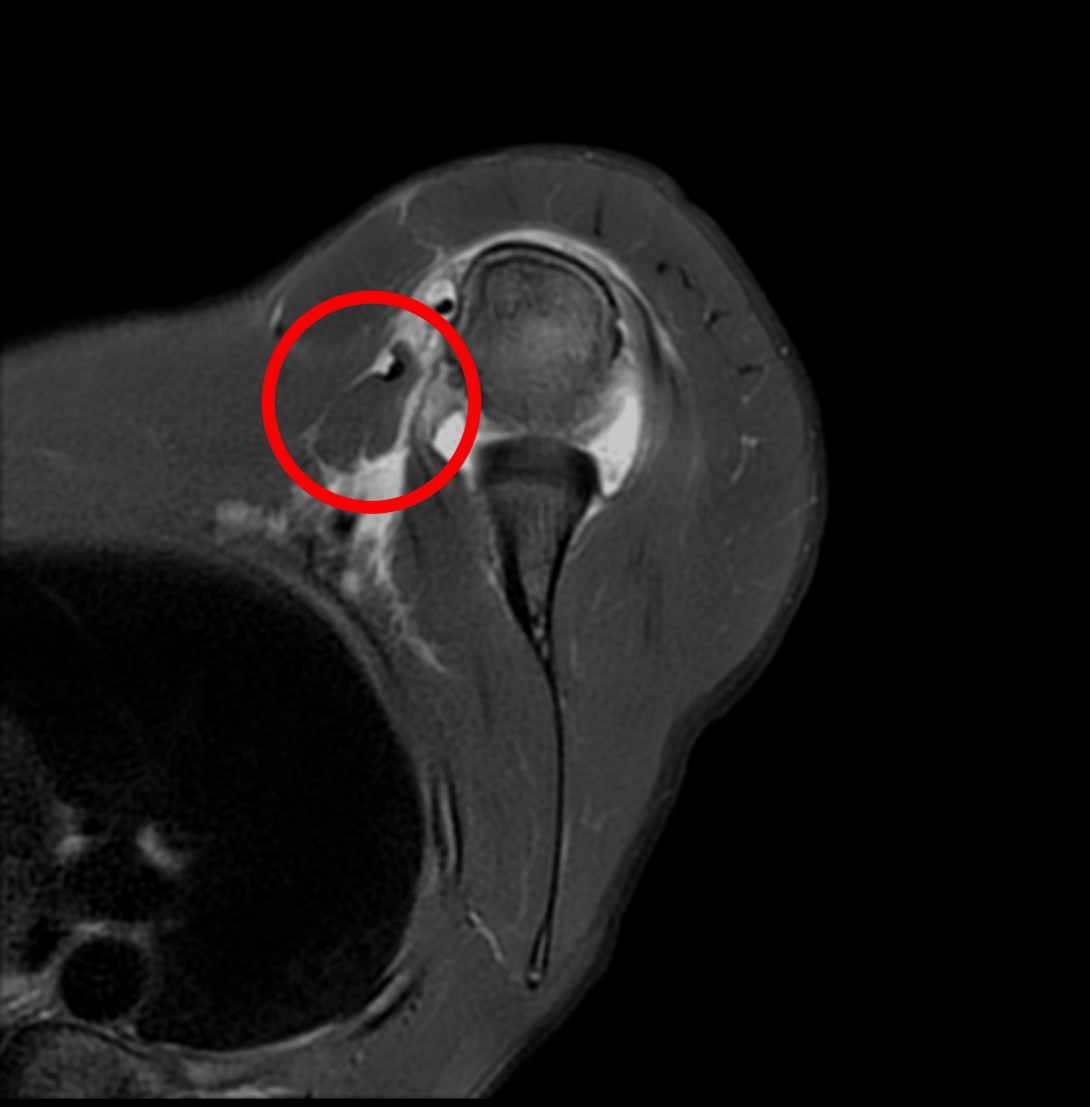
An axial MRI sequence shows a tear in the sternal head of the pectoralis major tendon.
